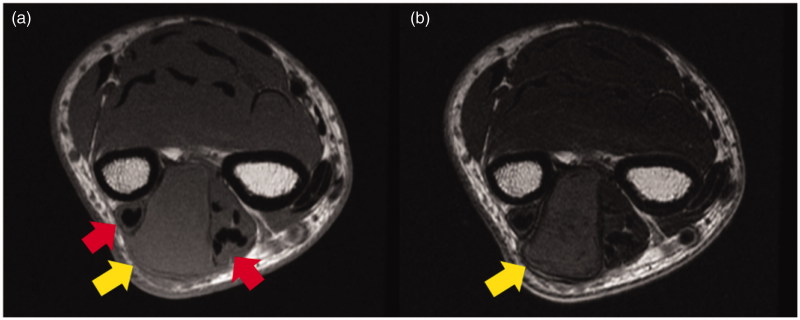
Preoperative MRI findings. (a) MRI (T1-weighed image): the mass was slightly hyperintense compared to the muscle and pushing outward against the extensor tendons (arrows). (b) MRI (T2-weighed image): the mass was slightly hyperintense compared to the muscle (arrows).
